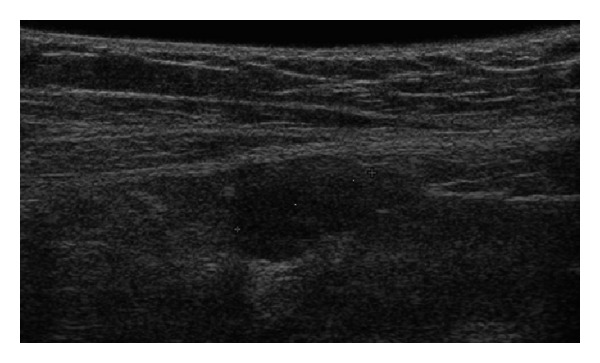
A 22-year-old girl/female with CAIS. Transabdominal ultrasonography (Hitachi EUB-8500, 14 MHz linear array probe) shows the presence of the right testis in the inguinal canal.
